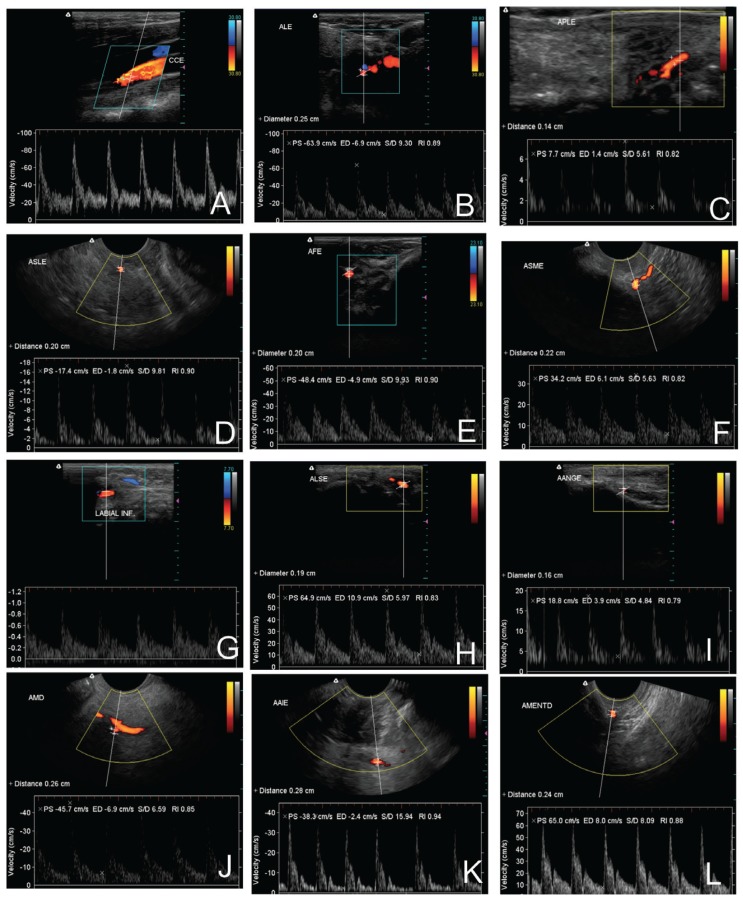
Ultrasonographies of the extraoral arteries: A-external carotid a., B- lingual a., C- submental a., D- facial a., E- inferior labial a., F- superior labial a., G- angular a., H- maxillary a., I- infraorbital a., J- temporal superficial a., K- transverse facial a., L-frontal a.
